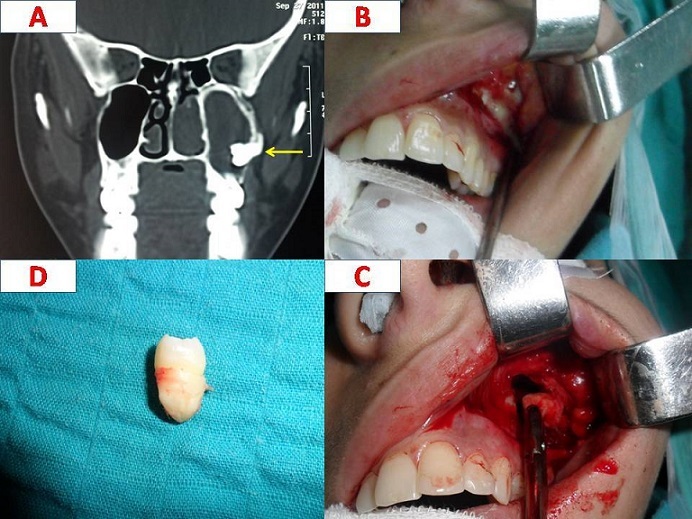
A): A coronal computed tomography (CT) of the paranasal sinuses in bone window revealing a filling of the left maxillary sinus byanhyperdense process attached to the outer wall: ectopic tooth; B): An operative view demonstrated the presence of an ectopic and supernumerary tooth in the maxillary sinus after incision according to Caldwell-Luc procedure; C): An operative view showing the extraction of the toothon the maxillary sinus; D): The extracted supernumeraryand ectopic tooth
